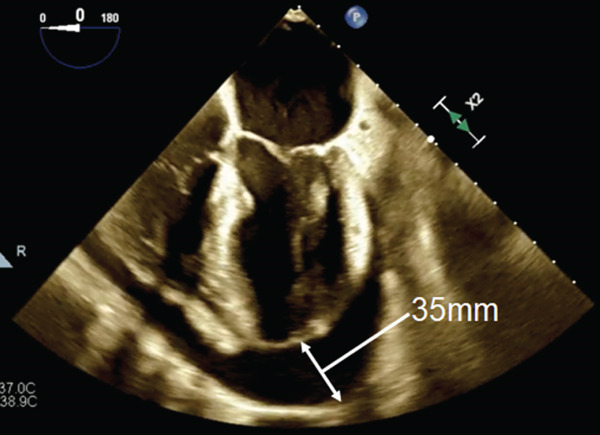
Transesophageal echocardiogram demonstrating a large pericardial effusion around the posterior left ventricle.
